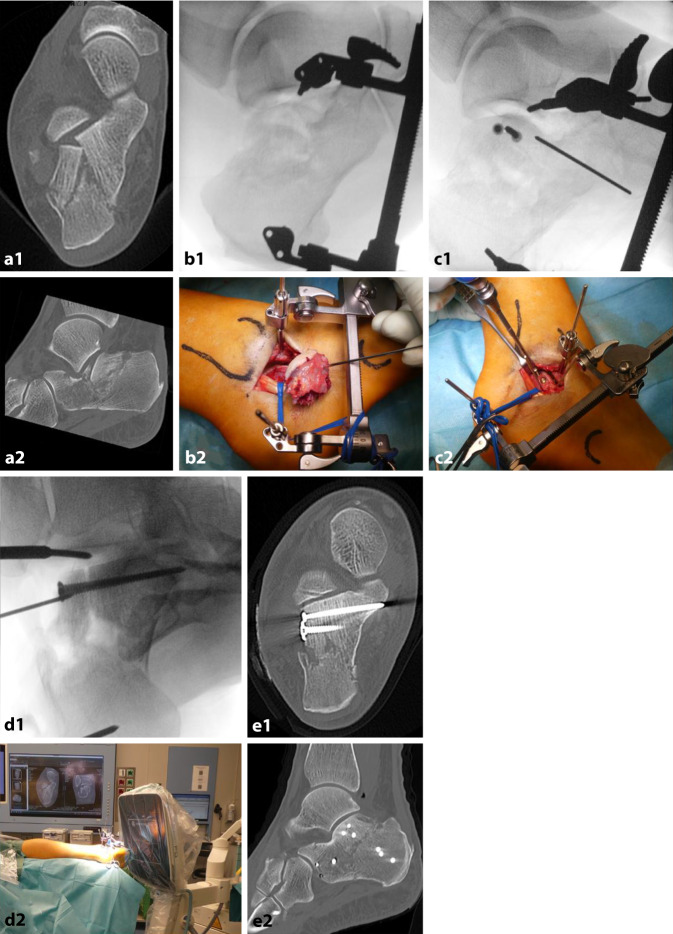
Klinischer Fall der Versorgung einer Calcaneus-Fraktur Typ IIA nach Sanders über einen Sinus-tarsi-Zugang mit primärer CT-Diagnostik in a_1_ parakoronarer und a_2_ sagittaler Rekonstruktion, Reposition des Tuber-Fragmentes mittels Distraktor im b_1_ seitlichen BV-Bild und b_2_ klinischem Situs mit Darstellung des lateralen Gelenkflächenfragmentes, Zugschraubenosteosynthese im c_1_ seitlichen BV-Bild bzw. im d_1_ Broden-View und jeweiliger klinischer Darstellung (c_2_ und d_2_) und postoperativem CT in e_1_ parakoronarer und e_2_ sagittaler Rekonstruktion
